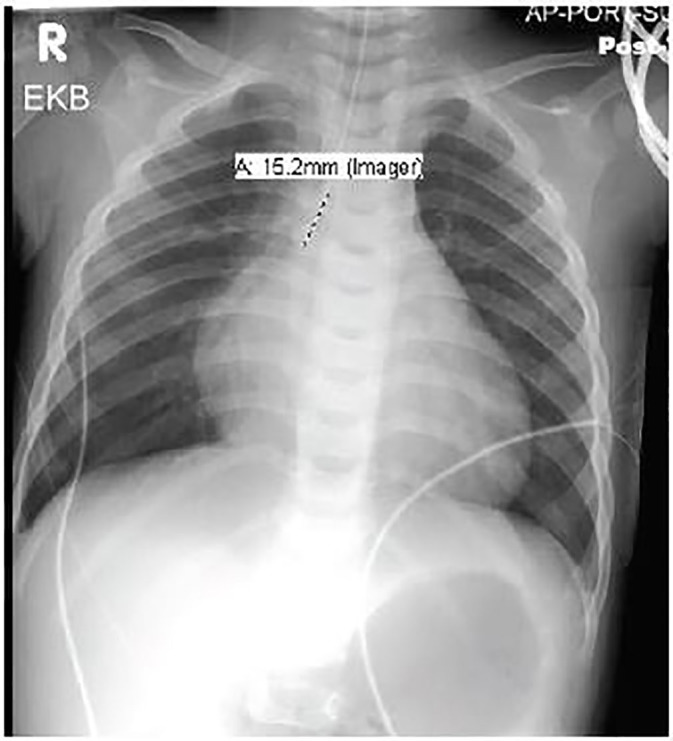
Post-intubation chest radiograph. Measurement indicates distance of endotracheal tube tip above carina.
